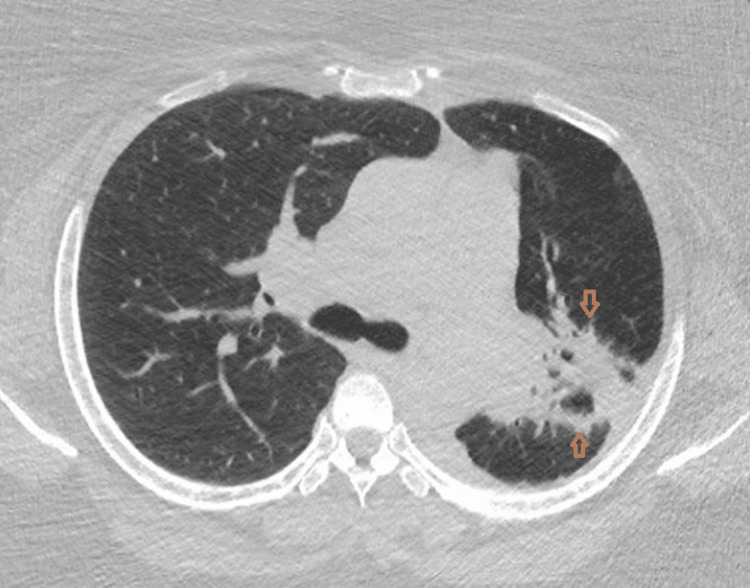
Axial lung window CT image showing dense left upper lobe consolidation with air bronchograms and ground-glass opacity abutting the mediastinum on the initial CT angiogram (arrows).
